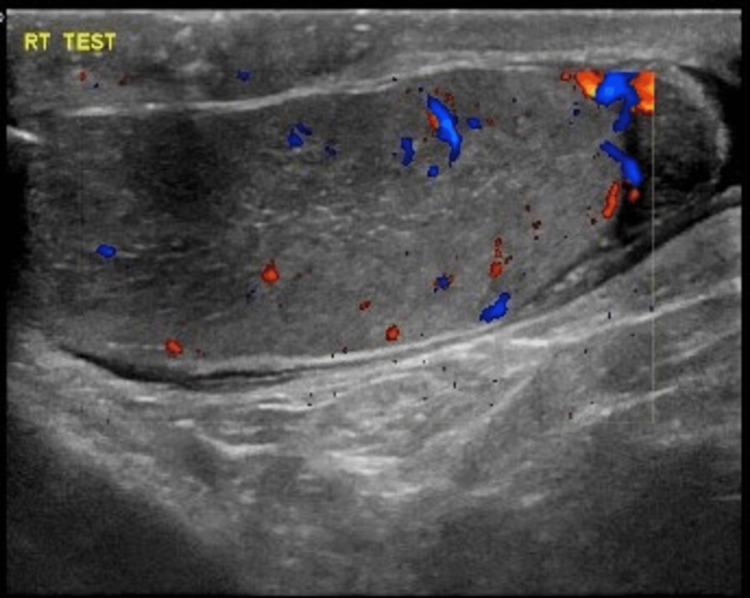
Postoperative scrotal Doppler ultrasonography displaying restored intratesticular vascularity on the affected side following detorsion and orchidopexy.
